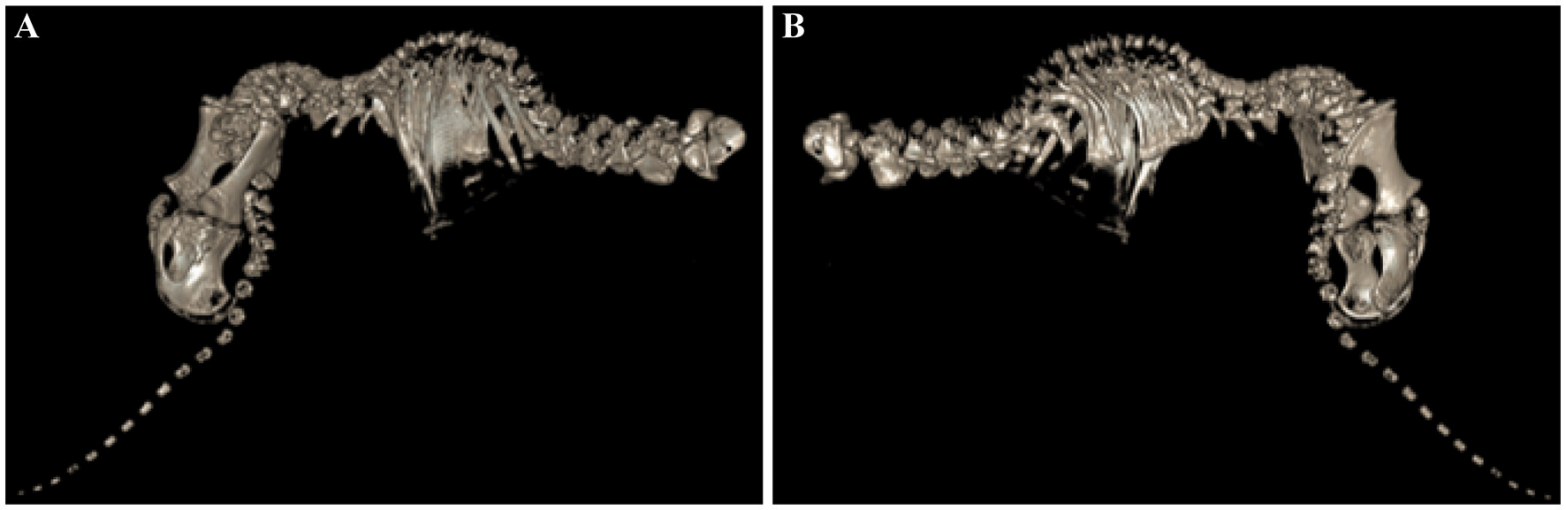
CT image of schistosomus reflexus of the fetus. A developmental defect characterized by a marked curvature of the spine and a deformed pelvis. (A) Kyphoscoliosis was mild in T1~11 and severe in L1~6; left view of the figure. (B) Vertebral dysplasia was observed in S1~5 and Cd 1~5; right view of the figure.
